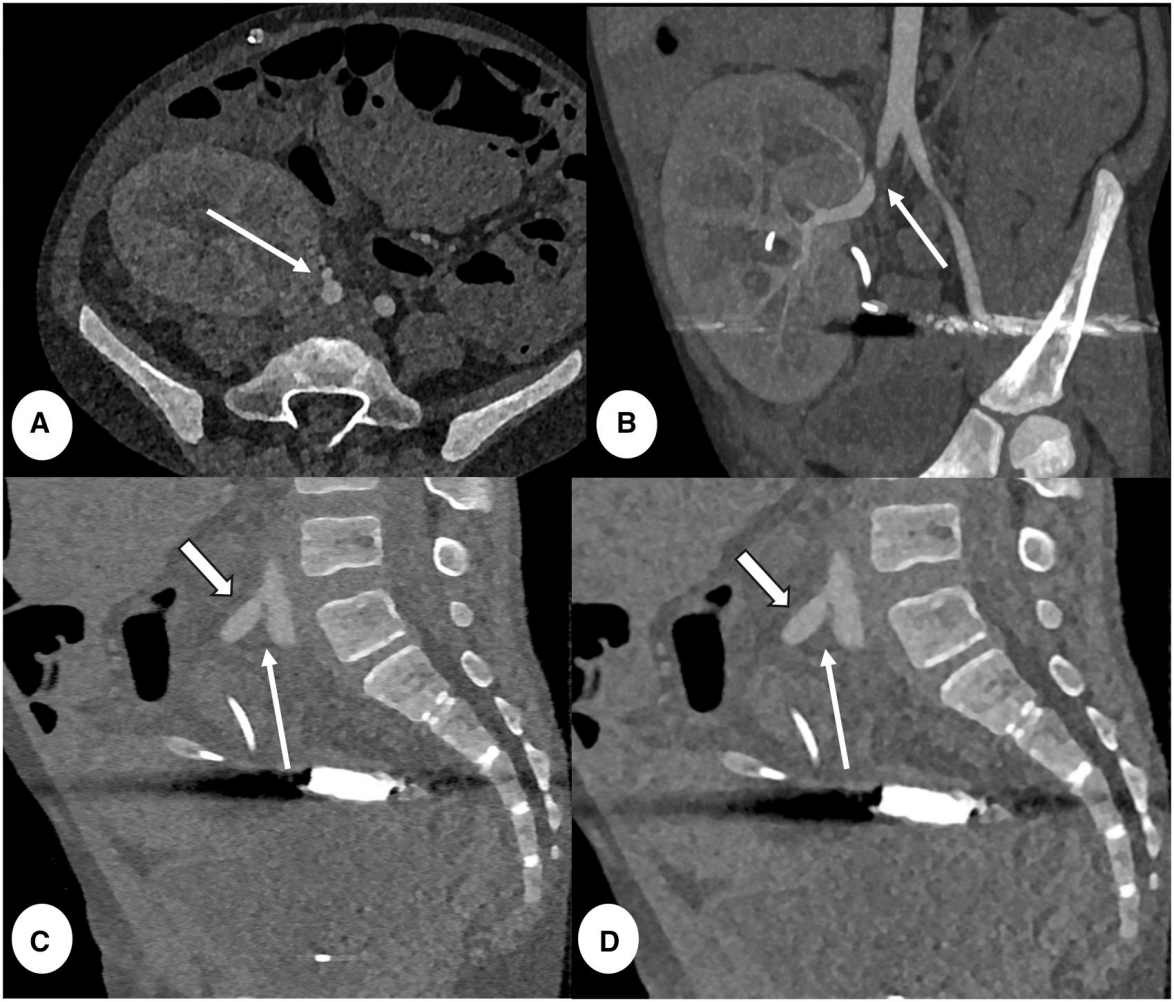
CT angiography abdomen in 9 years old male after kidney transplant complicated by renal artery anastomosis stenosis. (A) 0.2 mm axial, (C) 0.4 mm sagittal and (D) 0.6 mm sagittal PCD-CT images (reconstruction kernel Bv48 and IR level 4, Q4). (B) A 6 mm curved maximum intensity projection (MIP). Note lumen stenosis at the level of the anastomosis with the right common iliac artery (thin arrow A-D) and post-stenotic renal artery dilatation (thick arrow in C and D). CTA was conducted with a total dose-length-product (DLP) of 7 mGy×cm.
